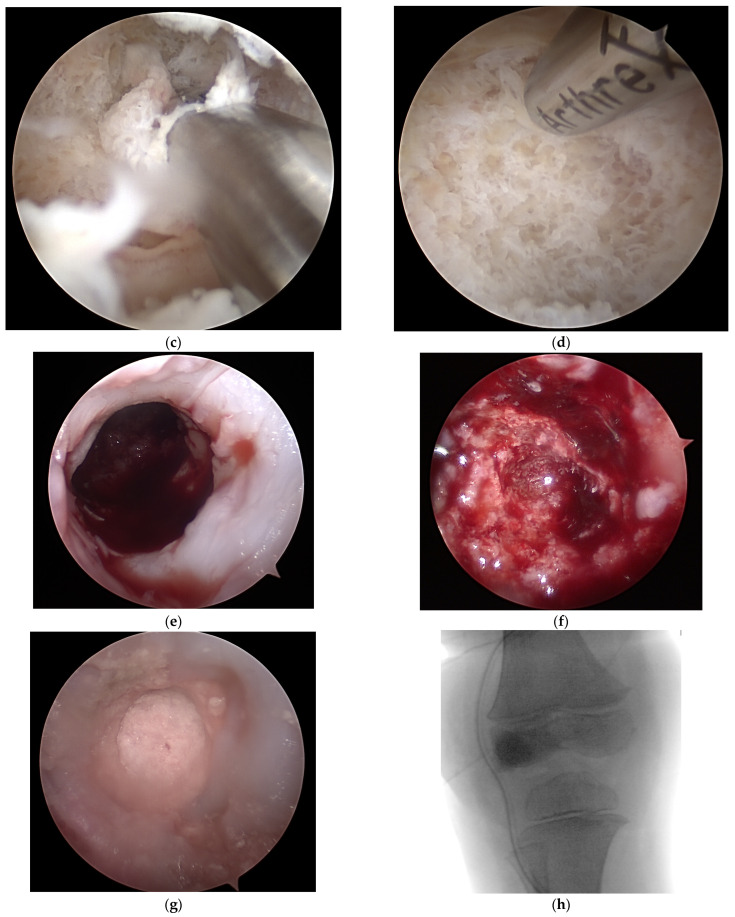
Synovitis and fibrinous deposition were observed in the lateral gutter of the knee joint (a), debris in the defect was identified (b) and removed with an arthroscopic shaver (c), until healthy tissue remained (d). The opening of the lesion to the lateral gutter (e) and the cavity surrounded by vital bone (f), filled by synthetic bone graft (g) and complete void filling, was also documented radiographically (h).
